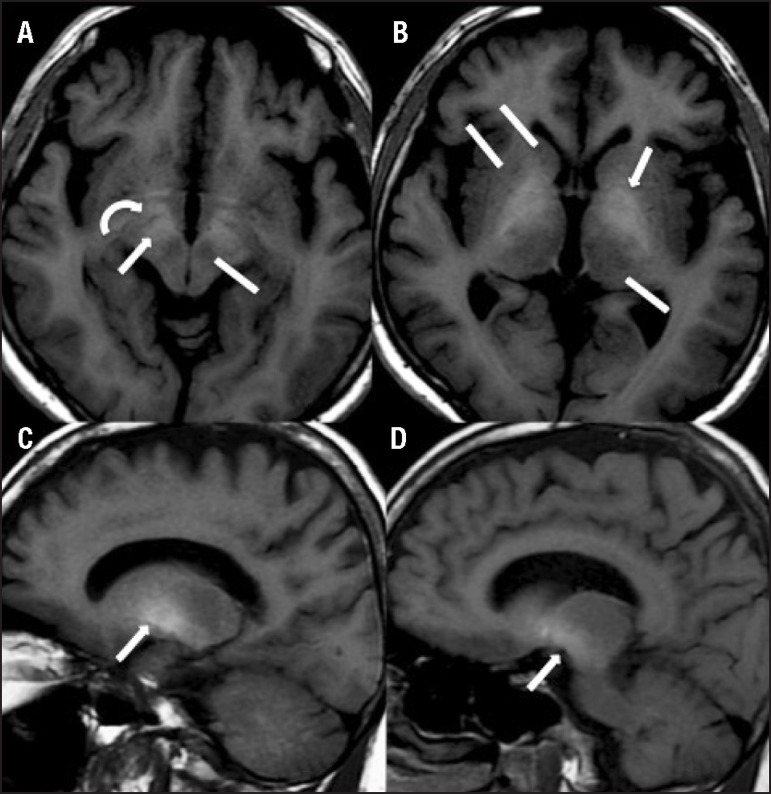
[A,B] Axial T1-weighted magnetic resonance imaging shows
